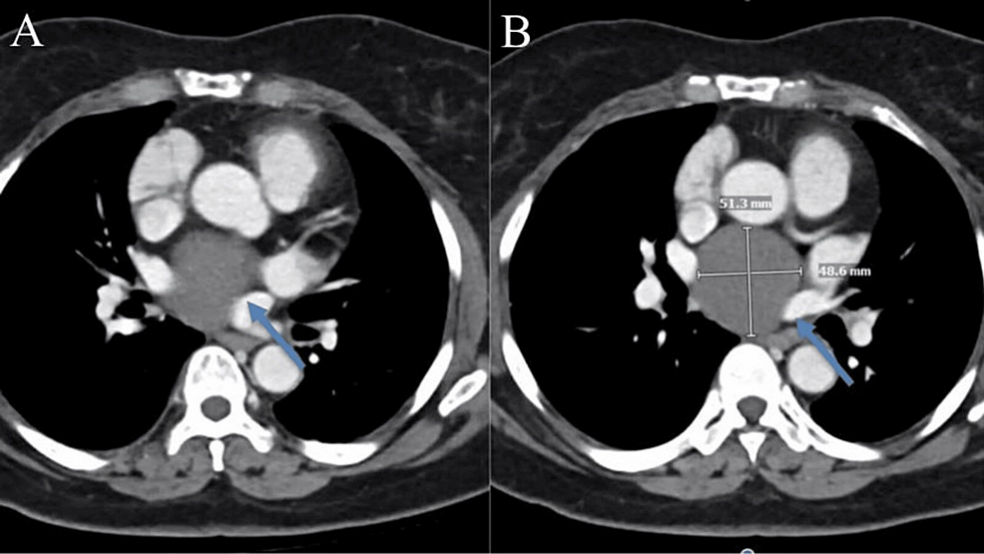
CT scans of the mediastinum and pulmonary hila, showing no lymph node enlargement in the axilla, supraclavicular area, mediastinum, or hilar region. A subcarinal low-density lesion measuring 5.1 × 4.9 cm (A) is observed, which was previously measured as 4.9 × 4.9 cm. No suspicious internal enhancing nodules are detected (B). CT, computed tomography
